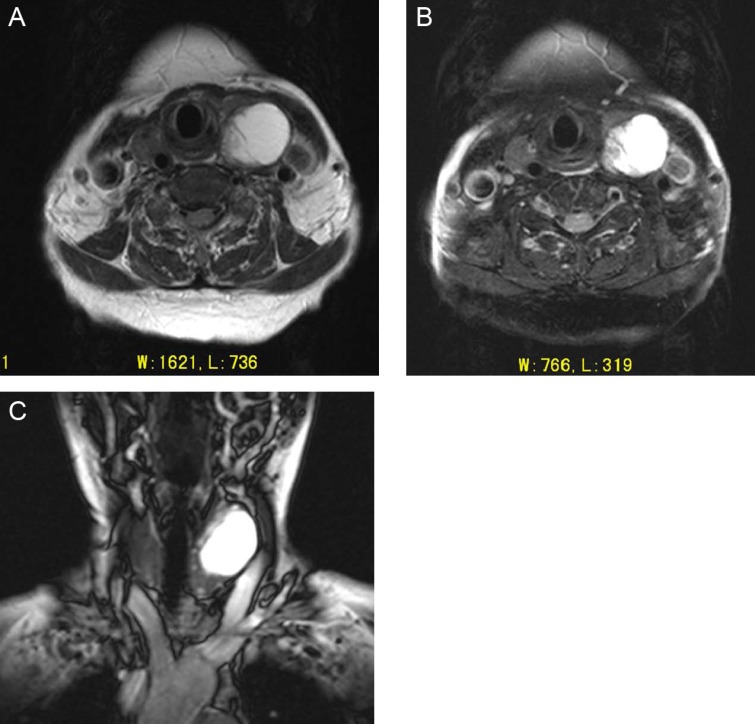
The cyst is located at the lower part of anterior neck behind the left lobe of the thyroid gland. High-intensity imaging of the content on both T1- (A) and T2- (B and C) weighted MRI suggested that the cyst area was filled with fluid.
